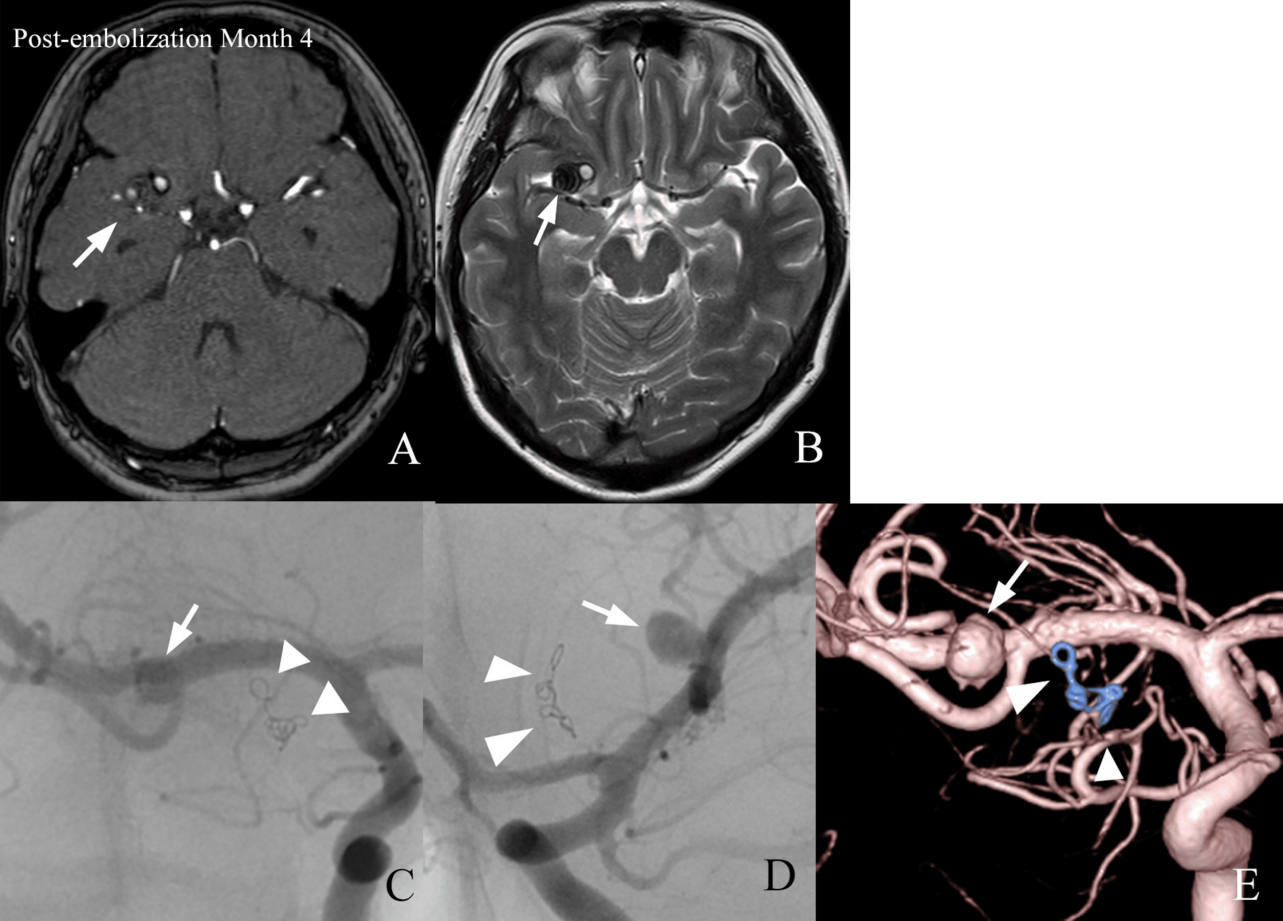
Follow-up imaging at four months after treatment (A) Time-of-flight magnetic resonance angiography and (B) T2-weighted imaging demonstrate an enlarged aneurysm measuring 12 mm in diameter, containing mixed high- and low-signal intensities suggestive of intraluminal thrombus (arrow). (C) Anteroposterior, (D) lateral, and (E) three-dimensional (3D) digital subtraction angiography (DSA) demonstrate further aneurysm enlargement, measuring 14 mm in dome diameter with a 4-mm neck (arrow). The coil mass is displaced anteriorly toward the non-opacified thrombosed portion of the aneurysm (arrowhead).
